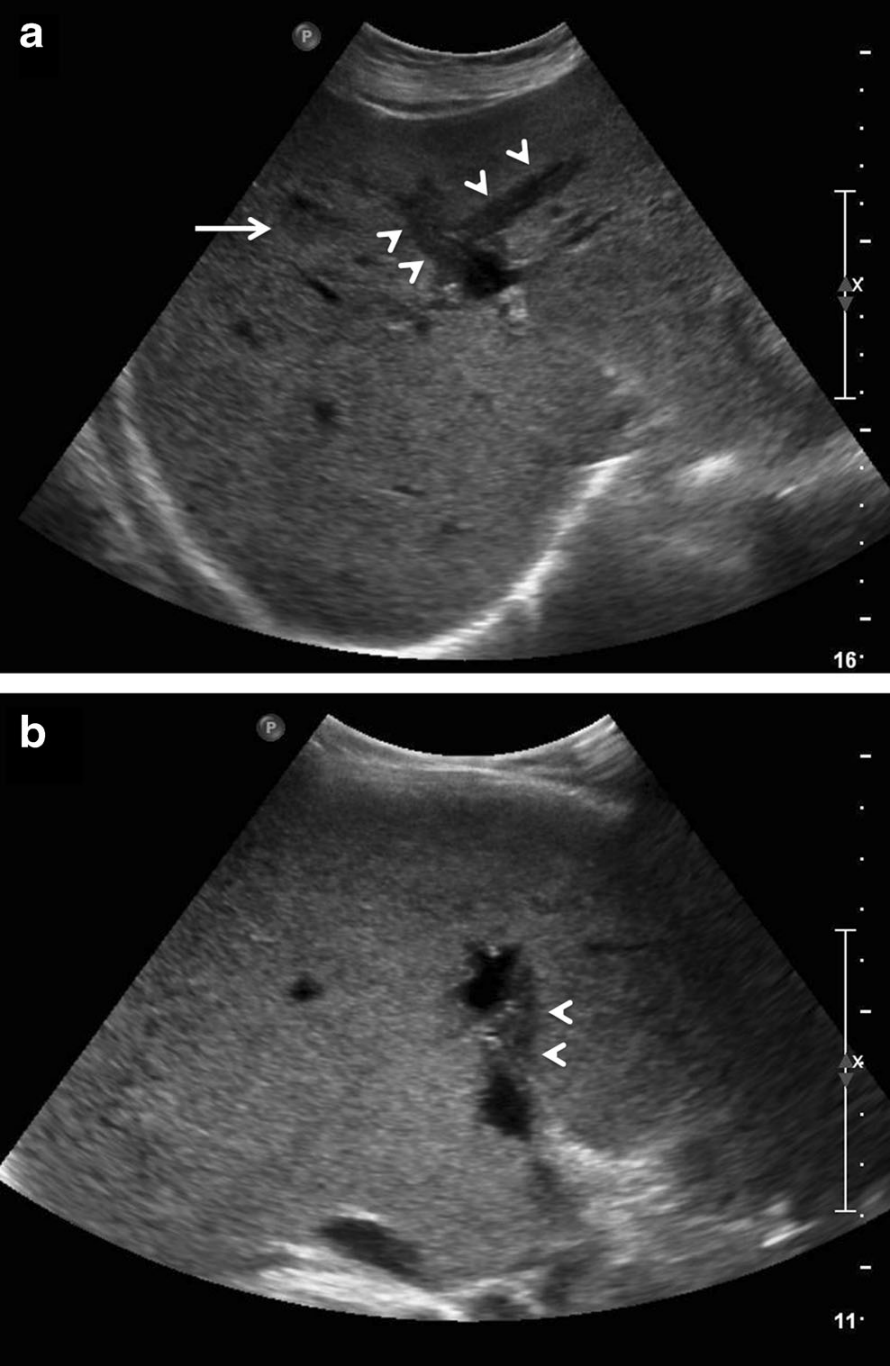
Non-Hodgkin lymphoma in a 34-year-old female with fulminant hepatic failure. Gray-scale US images demonstrating diffuse linear as well as nodular hypoechoic periportal cuffing (arrowheads and arrow, respectively). The patient had a rapidly downhill clinical course and succumbed to her illness within a week of admission. Post-mortem liver biopsy revealed findings consistent with diffuse large B-cell lymphomaMore commonly, however, a large seemingly infiltrating juxta-hilar mass is seen tracking along the biliary radicals and portal tracts. On US, the mass is usually hypoechoic. However, areas of heterogeneity as well as completely echogenic lesions have also been reported [49]. On CT, these masses are homogeneously hypodense and show poor enhancement (Fig. 15). Also, as previously described, they encase the portal vessels but do not occlude them, connoting their pliable nature and soft texture. Owing to its better soft tissue contrast, MR imaging can delineate the entire extent of these tumours, particularly when combined with diffusion weighted imaging. The severity of biliary dilatation can also be assessed simultaneously (Fig. 16).
